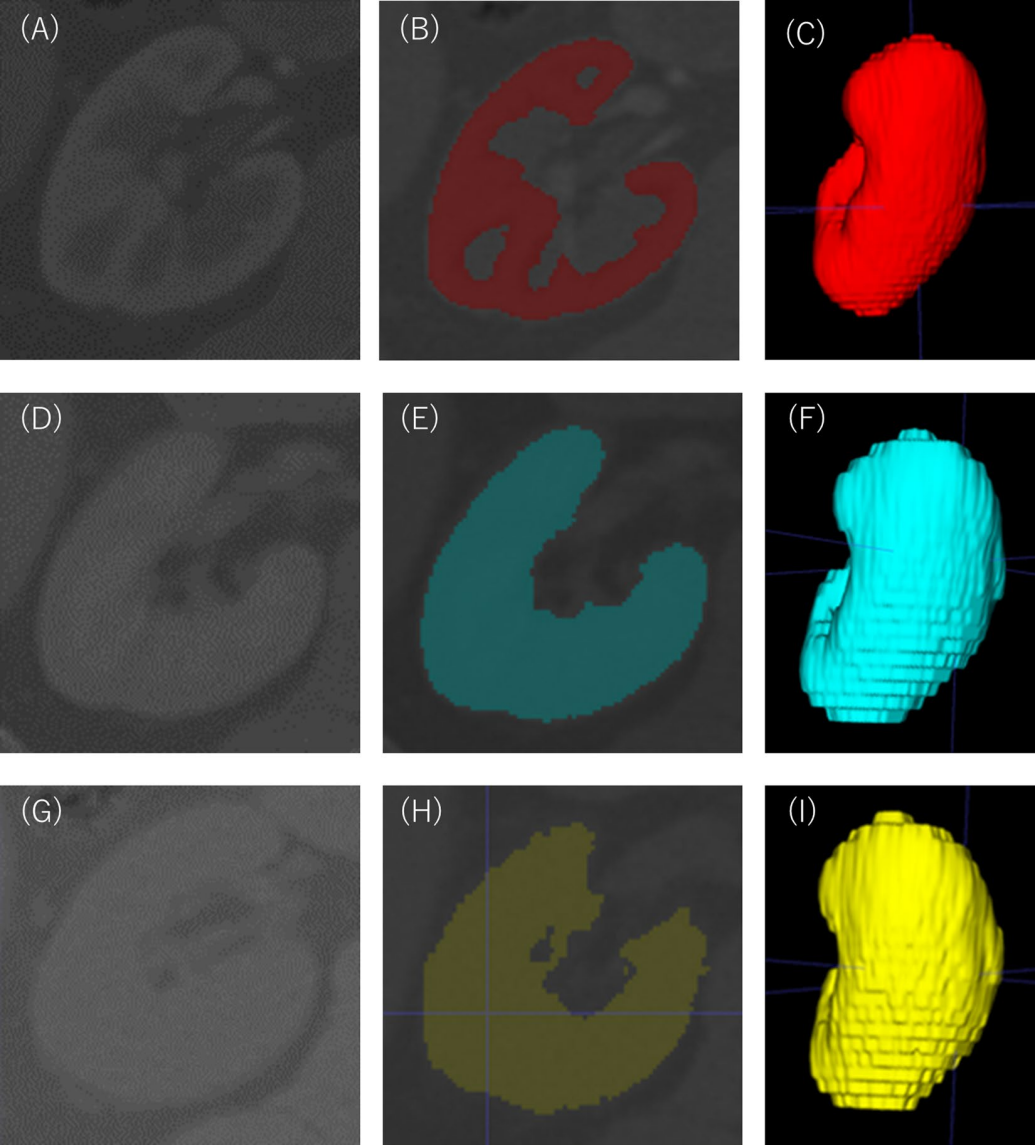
Measurement of renal cortical volume and renal parenchymal volume. A three-dimensional cortical image was semi-automatically constructed from the contrast-enhanced images obtained in the arterial phase after contrast media infusion (A–C). A three-dimensional parenchymal image was semi-automatically constructed from the contrast-enhanced images obtained in the venous phase after contrast media infusion (D–F). As with the venous phase, a three-dimensional image was semi-automatically constructed from the image of specific density areas of kidney obtained from unenhanced CT images (G–I). Renal artery, renal vein, renal pelvis, ureter, renal sinus, fat in renal sinus, adjacent tissue and adjacent organs were excluded from all the created images.
